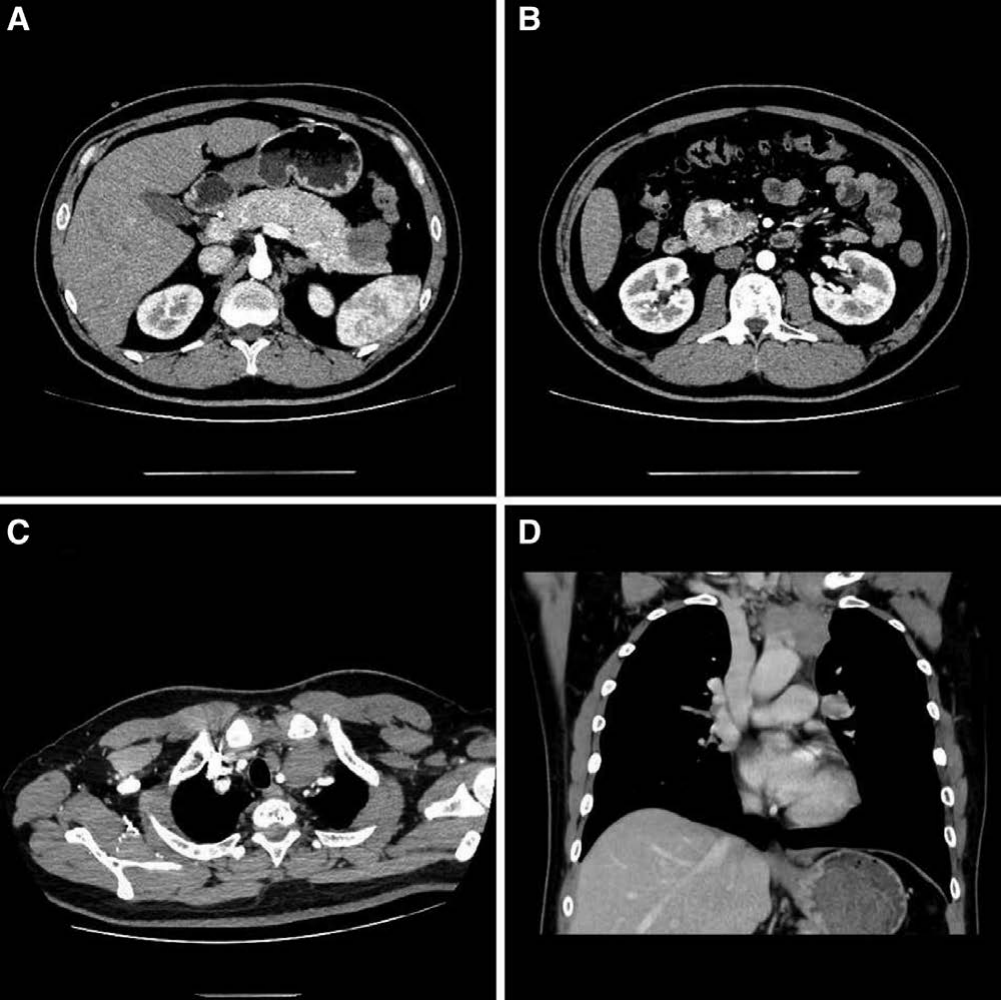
Contrast-enhanced computed tomography scan revealed: (A) Multiple lesions of pancreas. (B) Multiple enlarged lymph node shadows around the pancreas. (C and D) A mass of shadow in the anterosuperior mediastinum.
